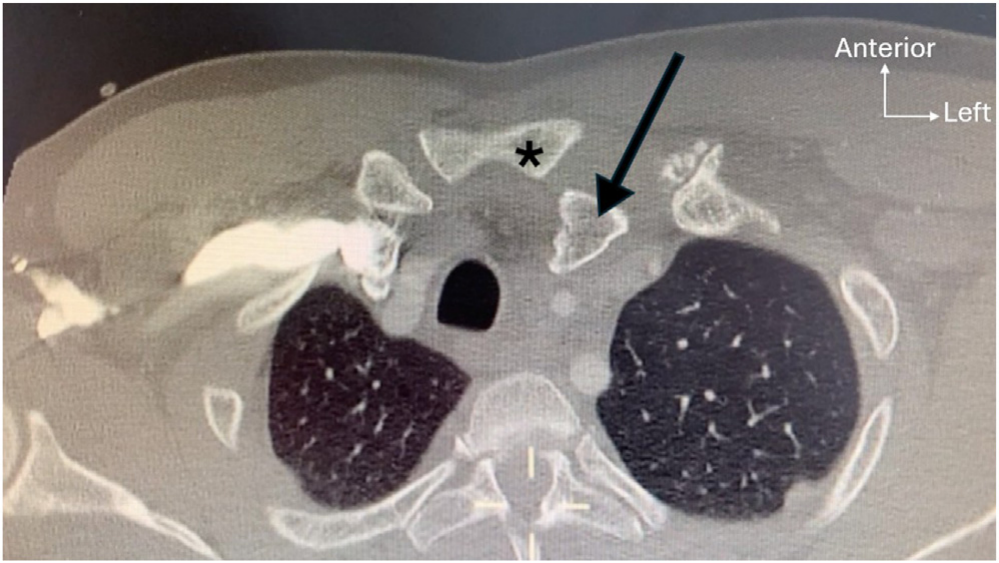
Axial computed tomography scan revealing a locked posterior dislocation of the left sternoclavicular joint. Medial left clavicle (black arrow); manubrium (asterisks).
